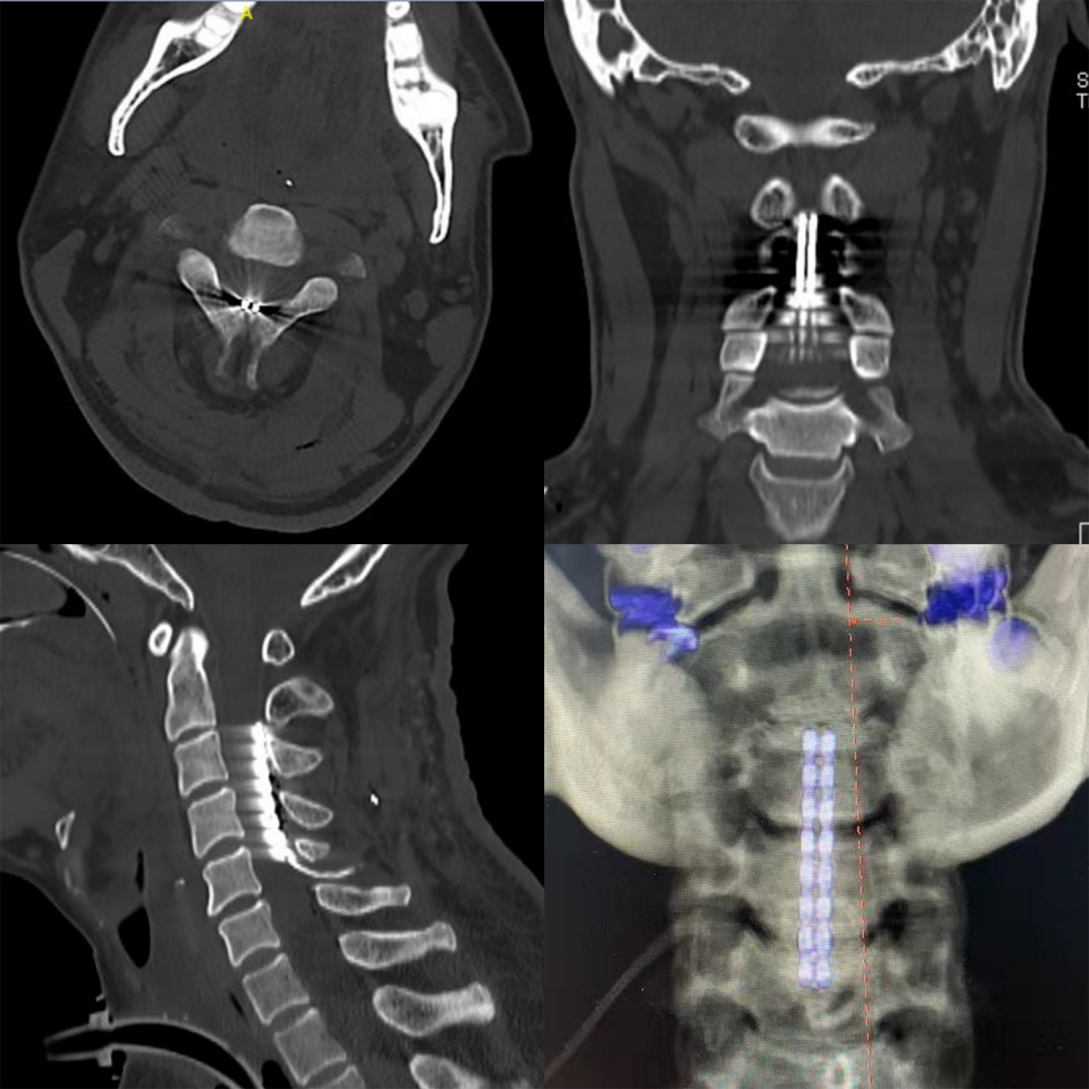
Cervical CT scan and reconstruction after SCS implantation. The electrode was implanted in the cervical spinal canal. Images 1–3 represent the axial, coronal, and sagittal views of cervical CT. Image 4 represents the sagittal view of VRT reconstruction
